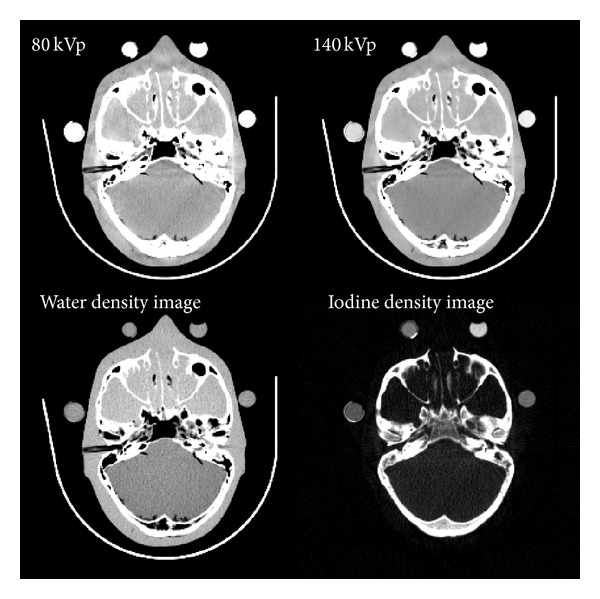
Exemplary reconstructed basis material density images, as well as the kVp images, from a dual-energy head CT exam.
